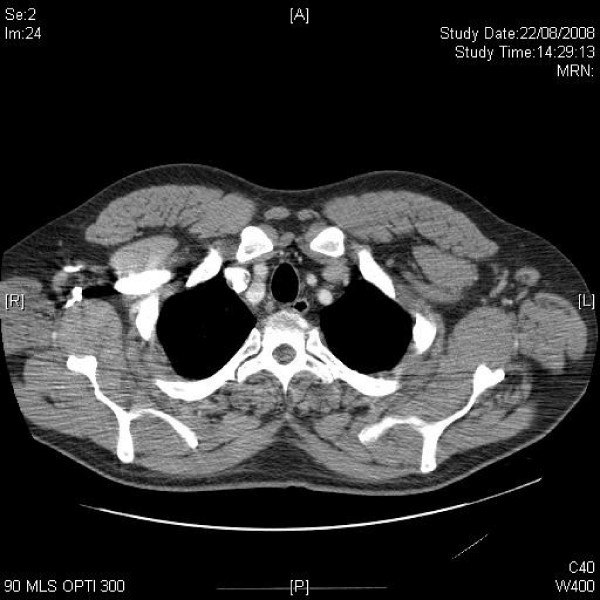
Axial CT of upper chest demonstrating axillary lymphadenopathy.
